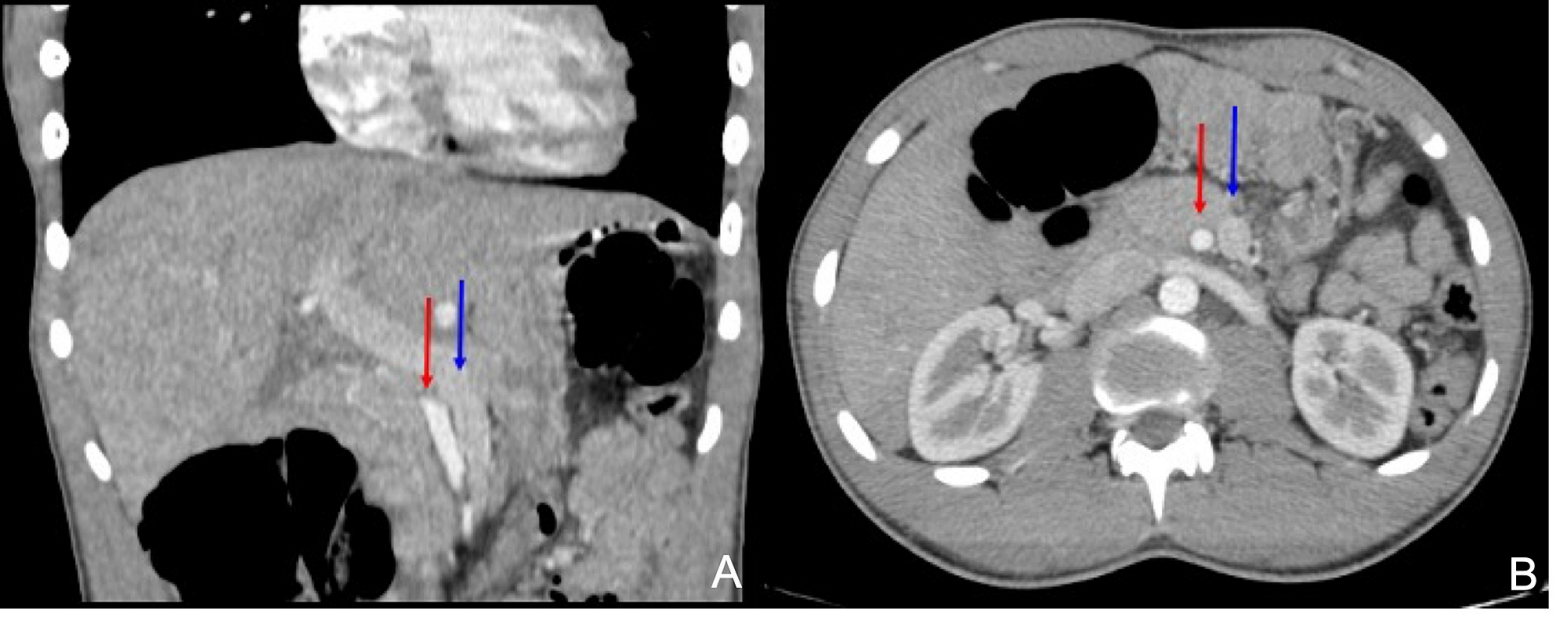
CECT view 2 Coronal (A) and axial (B) views demonstrating 'reversed positioning' of the SMA (red arrow) and SMV (blue arrow) to the left CECT: contrast-enhanced computed tomography; SMA: superior mesenteric artery; SMV: superior mesenteric vein
